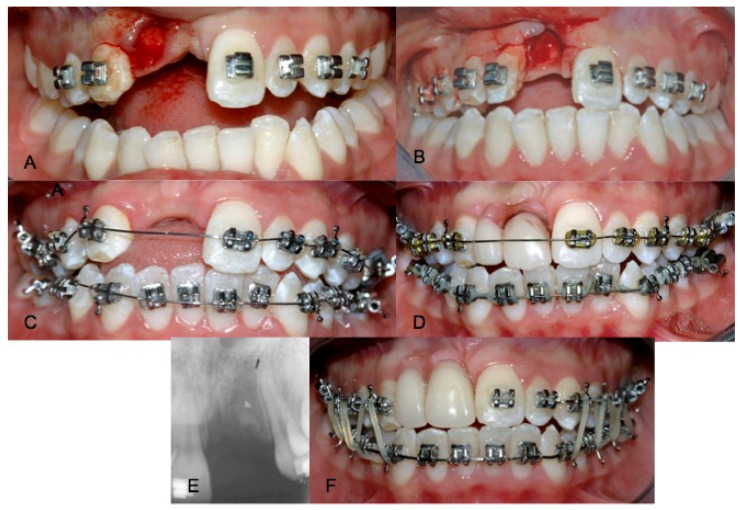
(A) Extraction of 12, (B) extraction of 11, (C) start of orthodontic therapy, (D) removable prosthesis placement, (E) periapical X-ray after two months and (F) orthodontic treatment after 4 months.
